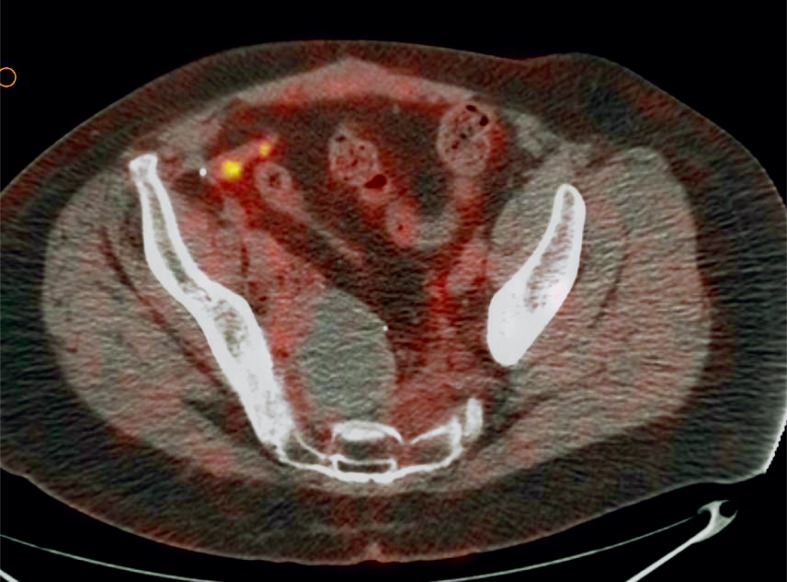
54 year old male with extraskeletal myxoid chondrosarcoma. FDG-PET/CT imaging shows mild peripheral FDG uptake with an standard uptake value (SUV)_max_ of 2.8 in a patient with mildly dense tumor burden.
